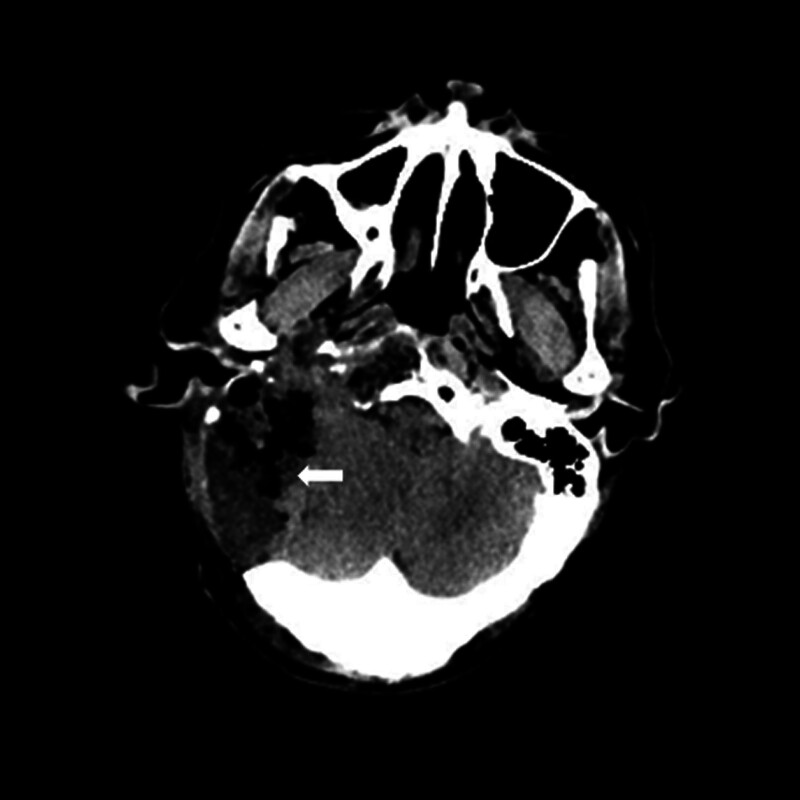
Postoperative computed tomography revealed partial loss of the right skull, with mixed density shadows and gas shadows in the operative area.
